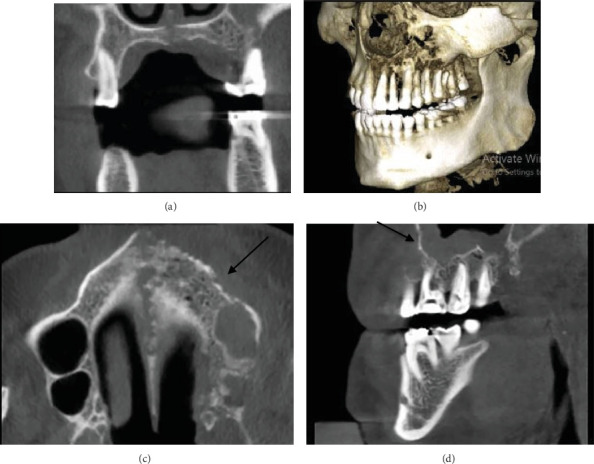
(a) Coronal CBCT view showing bone destruction, perforation of palatal bone, and obvious swelling of soft tissues in the left maxilla. (b) The reconstructed 3D image demonstrates the destruction of the bone and right maxillary sinus. (c) Axial CBCT view showing the involvement of the nasal bone and left sinus. The arrow shows the cortical bone destruction. (d) Sagittal CBCT view with the arrow showing the mucosal thickening of the sinus and bone destruction around the molar teeth.
